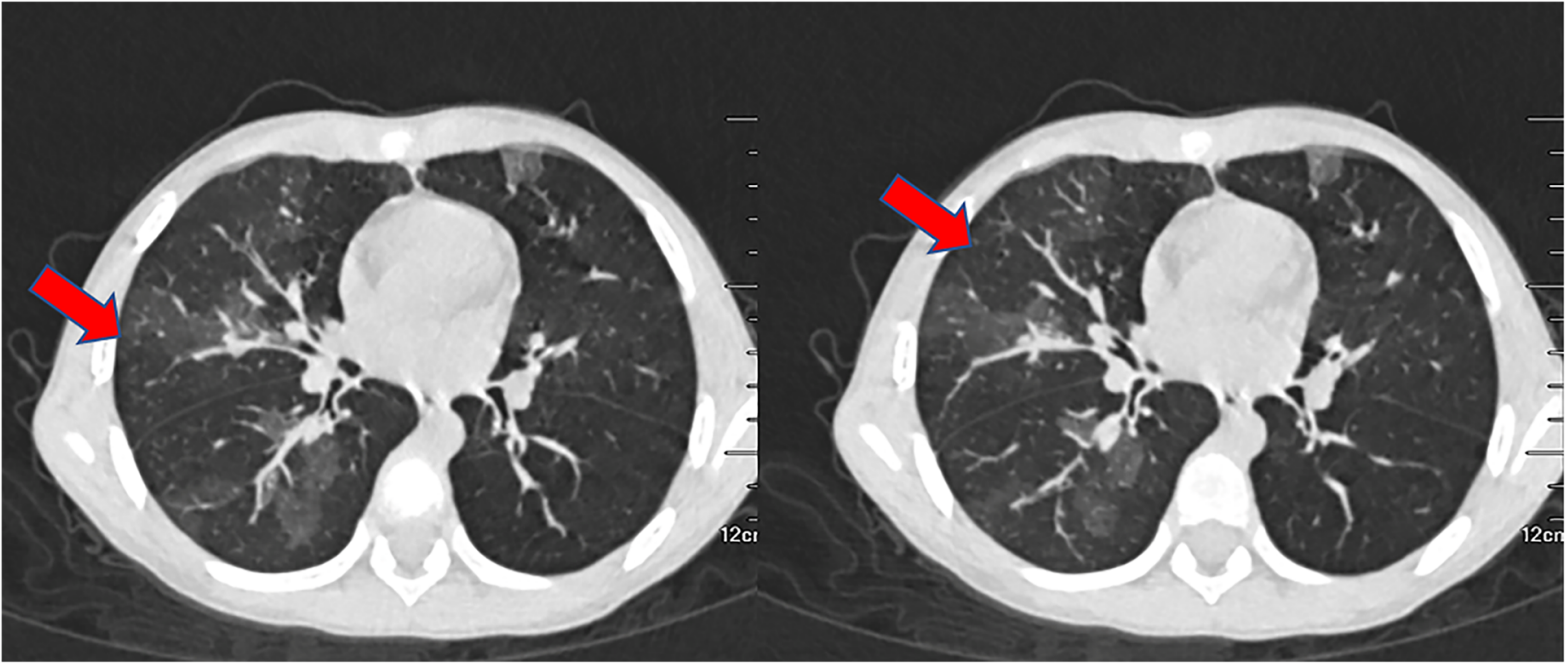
The CT scan of the chest performed 43 days after admission displayed widespread mosaic pattern.
